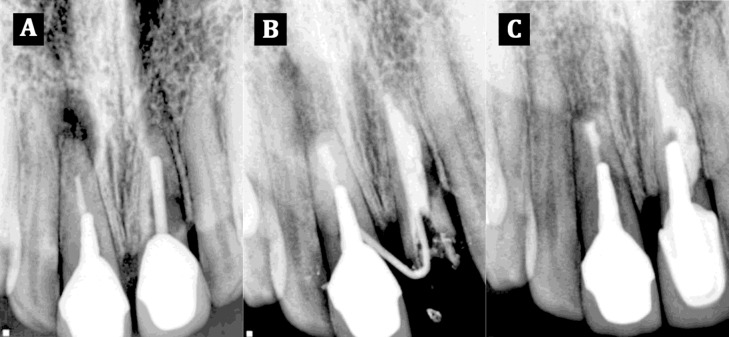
A) Preoperative radiograph; B) Root filling radiograph; C)Eighteen months follow-up radiograph
